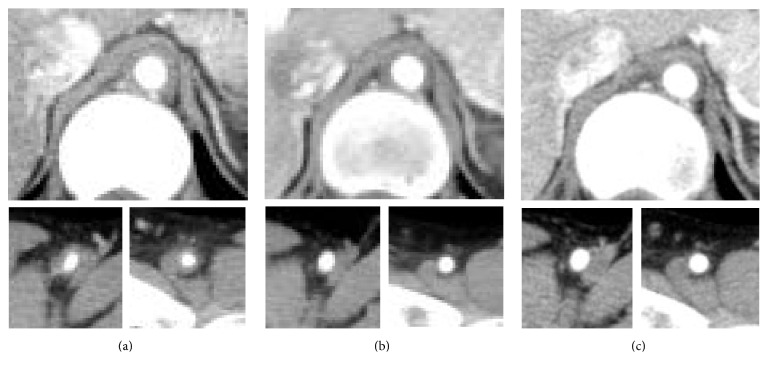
Changes in the computed tomographic findings of arterial lesions of Takayasu arteritis before and after starting tocilizumab therapy. Contrast-enhanced CT of the descending aorta (the upper), the right femoral artery (the bottom left), and the left femoral artery (the bottom right) was performed. Three months before starting tocilizumab therapy, while on the treatment with infliximab, arterial wall thickening of the femoral arteries was observed (a). After six months of tocilizumab therapy, there was a significant decrease in the wall thickness of the descending aorta and the femoral arteries (b). After 17 months of tocilizumab therapy, there was a further decrease in arterial wall thickness and an increase in the intraluminal diameter of the descending aorta and the femoral arteries (c).
